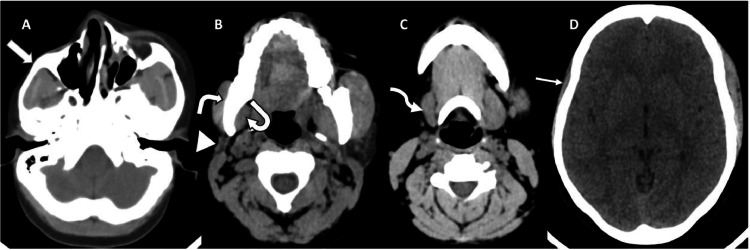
(A-D) Axial head CT scan (soft tissue window) showing thinning of the subcutaneous fat and skin over the right malar region (*block arrow*) (A) as well as atrophy of the right parotid gland (*arrowhead*) (B), right submandibular gland (*squiggle arrow*) (C), right masseter muscle (*white bent arrow*) (B), right medial pterygoid muscle (*curved arrow*) (B) and right temporalis muscle (*straight arrow*) (D).
